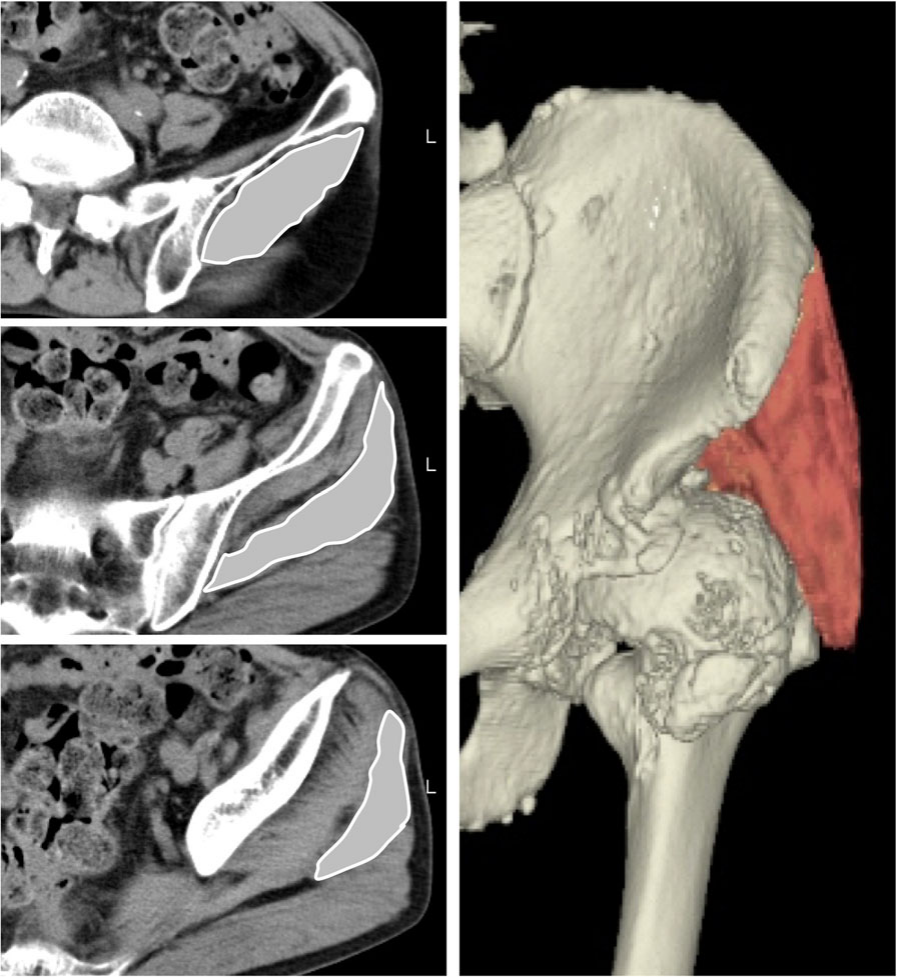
Muscle volume and fatty degeneration of the gluteus medius (GM). By tracing the cross-sectional areas of the GM on every third slice of the CT images using three-dimensional (3D) image analysis software (stripe area), GM muscle was reconstructed three-dimensionally (colored area). Muscle volume and fatty degeneration of the reconstructed GM were quantified using dedicated software
